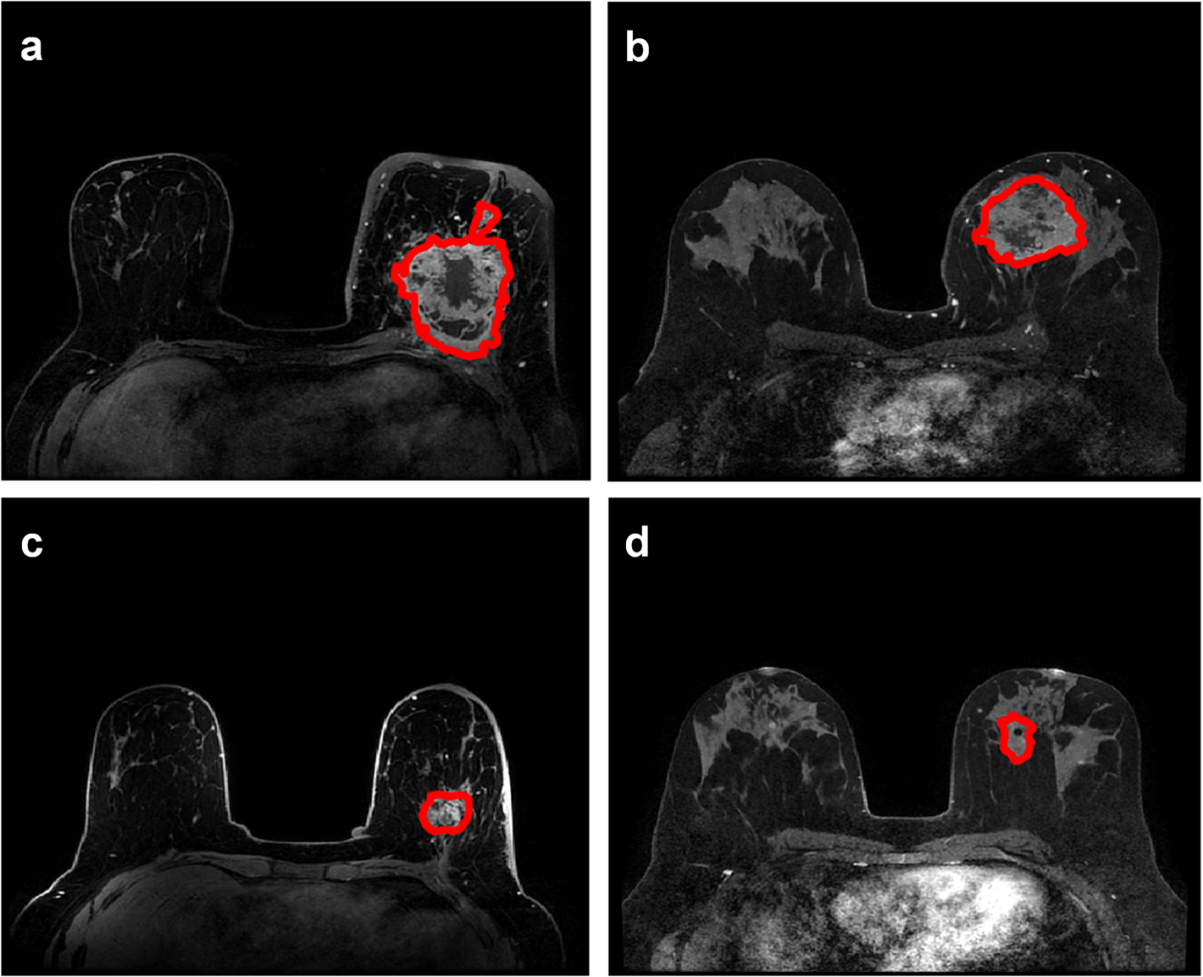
Representative pre-neoadjuvant chemotherapy (NAC) fat-saturated first post-contrast MRI (a and b) and post-NAC fat-saturated first post-contrast MRI demonstrating c no pathologic complete response (no pCR) for a and d pCR for b
